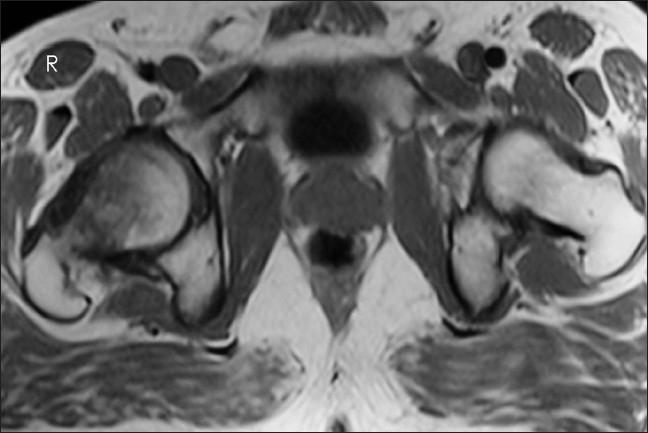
T1-weighted transverse image showing the bone marrow edema head and neck of right femur
